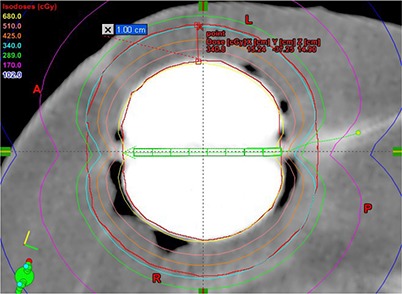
Two‐dimensional CT image to show a typical MammoSite balloon with isodose distribution for patient 19. The volume of PTV was the same with PTV_EVAL for this patient because the balloon was located in the middle of breast and the volume of skin +5 mm and lung/pectoralis muscle did not interfered with the volume of balloon +1 cm. Eight possible dwell positions were used along the straight MammoSite balloon catheter.
